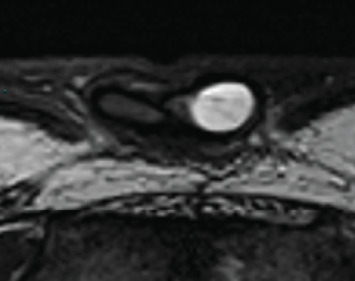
MRI of the penis: a well-circumscribed multilocular cystic structure with enhancement of the septa located at the laterodorsal part of the base of the left corpus cavernosum.
